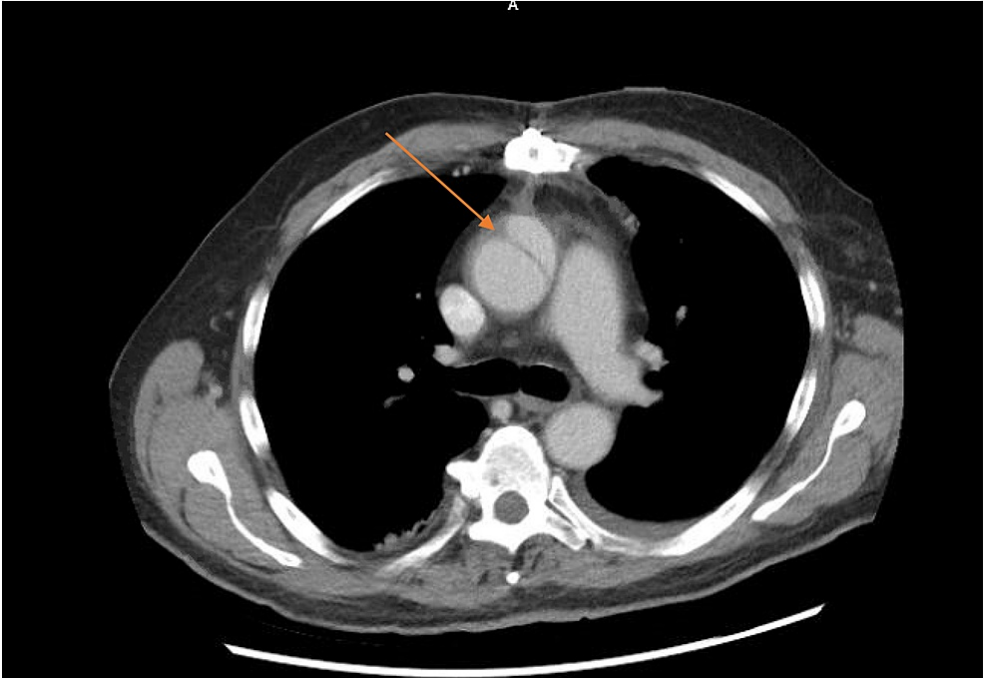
CT chest demonstrating a new dissection of the ascending thoracic aorta with false lumen adjacent to the true lumen. Arrow in figure pointing to dissection.
